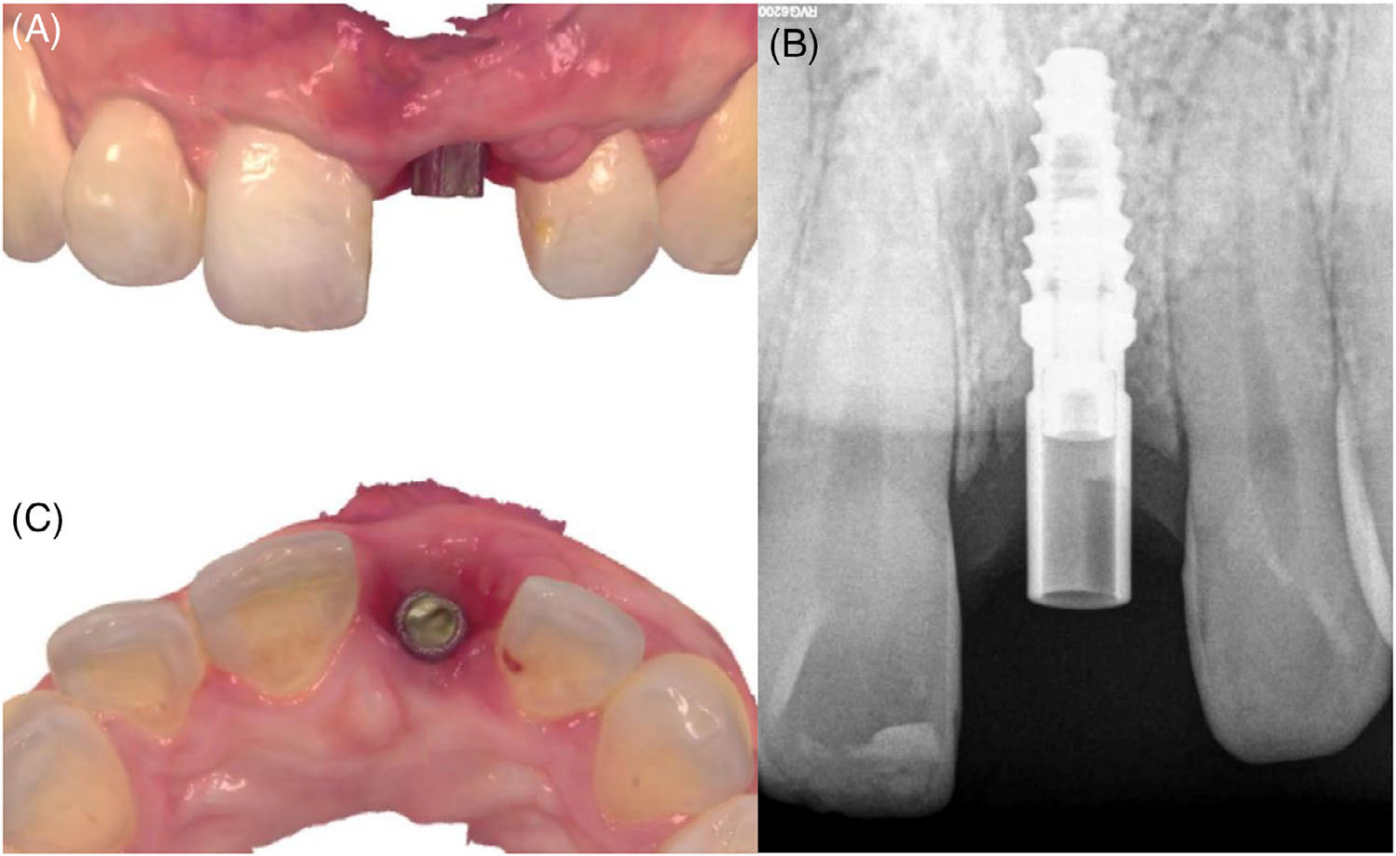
Digital impression. Frontal view of the intra‐oral scan (A). Occlusal view of the intra‐oral scan (C). Periapical radiograph (B).
